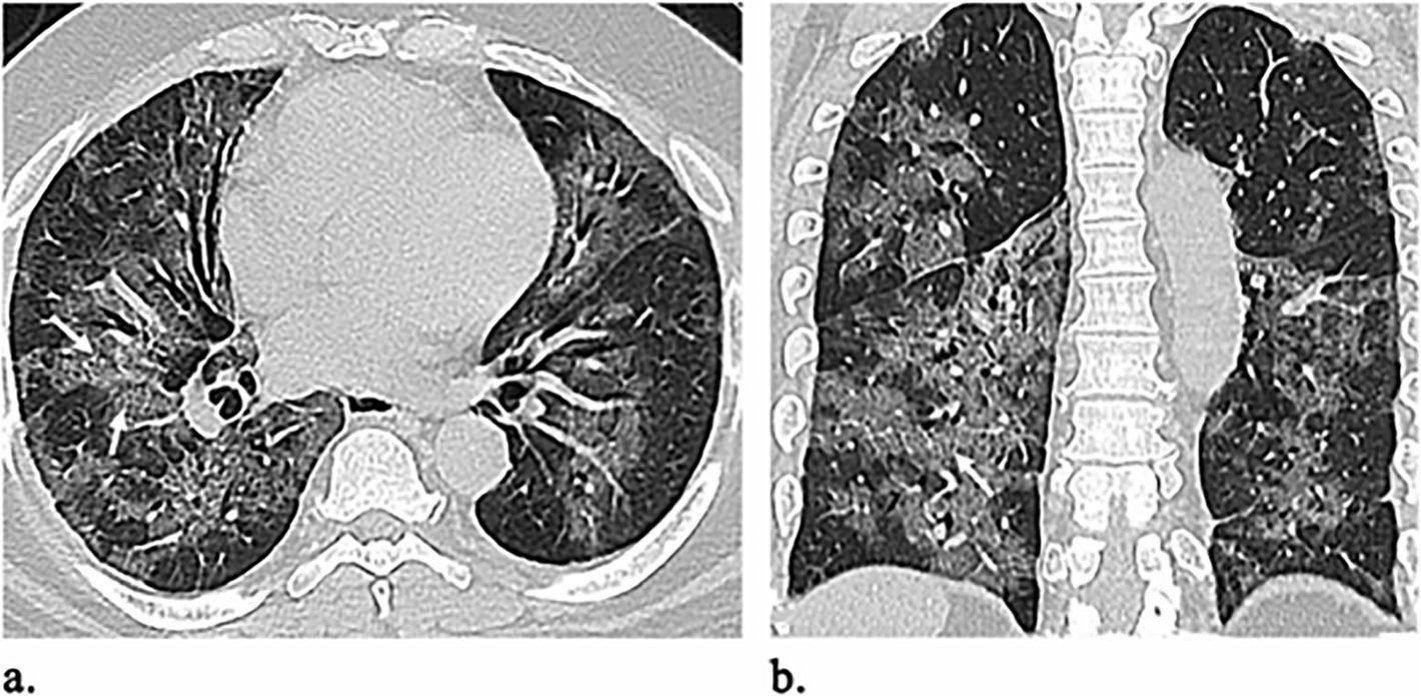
A 49-year-old female patient presented with epigastric pain, diarrhea, cough, and dyspnea. O_2_ saturation was 90%. a Axial and b coronal unenhanced HRCT images show bilateral upper and lower lobes extensive parenchymal involvement by GGO with intralobular septal thickening (white arrows) resembling crazy paving distributed in the hilar, centrilobular, and subpleural regions. No definite consolidations
